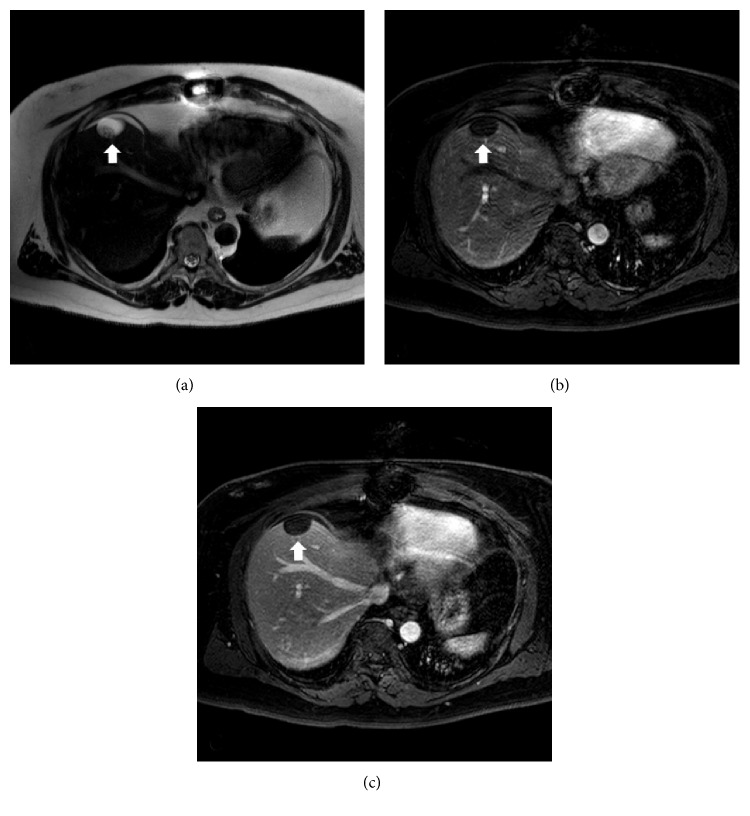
Transverse abdominal MRI of hepatic cyst. (a) T2-weighted image. (b) Arterial phase following intravenous contrast. (c) Venous phase following intravenous contrast.
